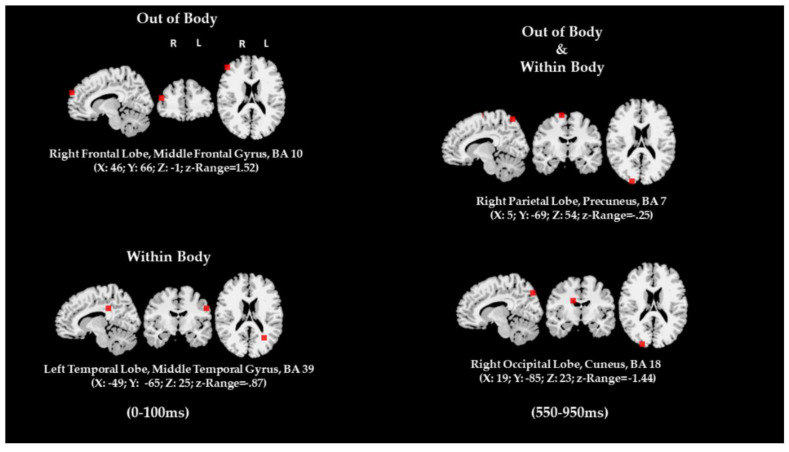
Vivid imagery experienced outside and within the body shows both similar and distinct loci of activation in the brain. Figure taken from D’Angiulli et al. [77]. Red points indicate the estimated anatomical graphic location of the generating source of EEG activity on the structural MRI template slices (i.e., sagittal, coronal, axial); the formal label, Talairach coordinates, and millisecond detection time intervals are given below the template according to Broadman Areas (BA).
